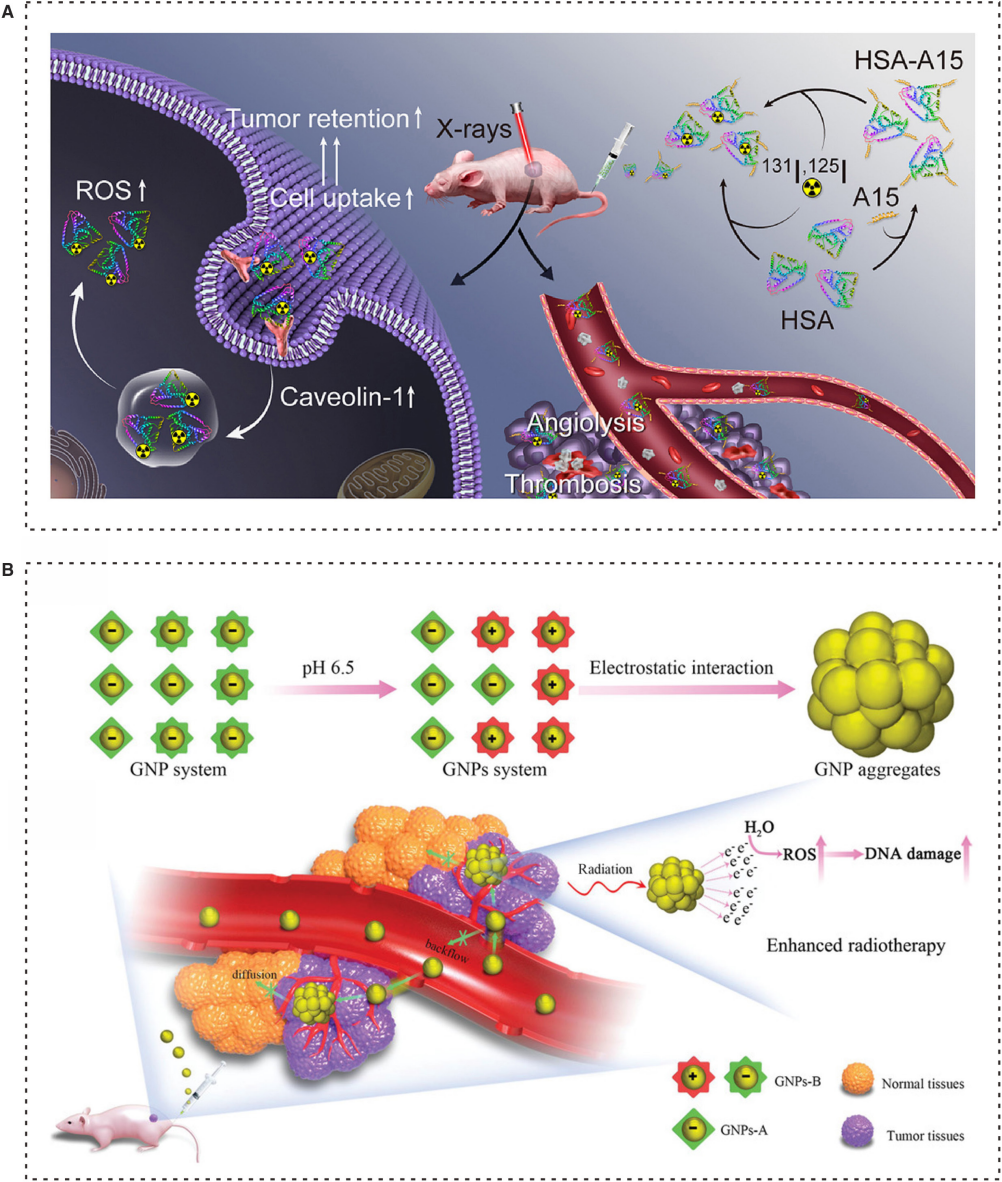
Nanotechnology enhances RT sensitivity. (A) X-ray irradiation increases expression of caveolin-1 and enhances RT sensitivity by promoting cellular uptake of HSA NPs and prolonging tumor retention. Adapted with permissions from [75]. Copyright 2020, Elsevier. (B) Acid-triggered small-size GNPs accumulate within tumors and act as tumor radiosensitizers by generating more ROS to damage DNA. Adapted with permissions from [87]. Copyright 2019, John Wiley and Sons.
